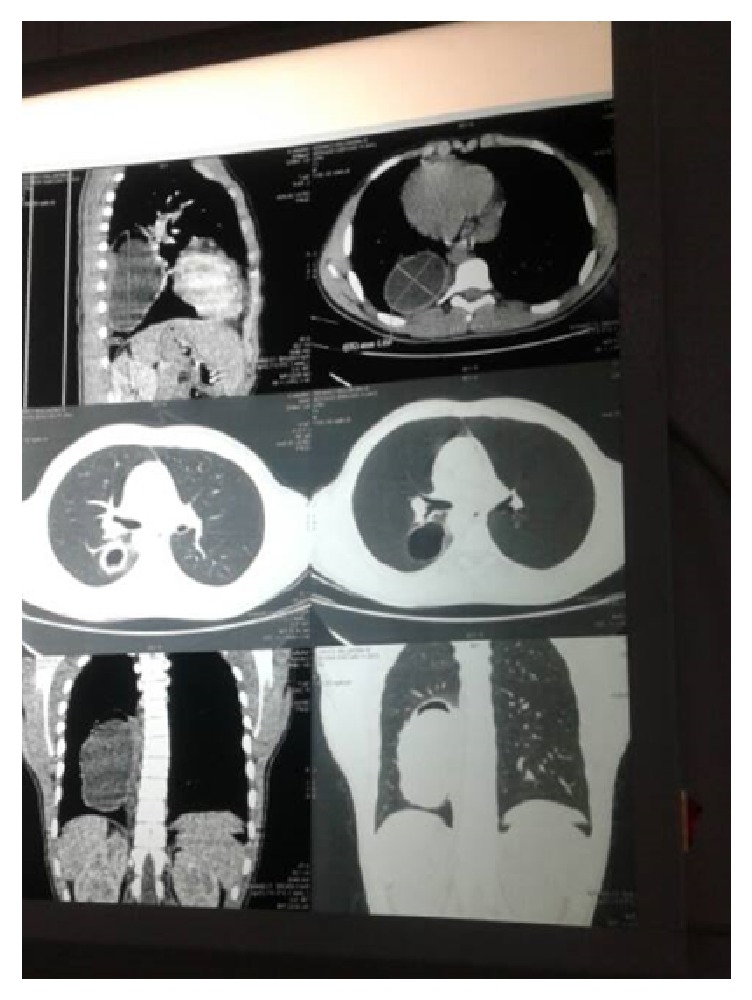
Chest CT scan with a well-circumscribed fluid attenuation lesion with homogenous content and smooth, hyperdense walls located in the lower lobe of the right lung measuring 30 by 20 by 27 cm.
